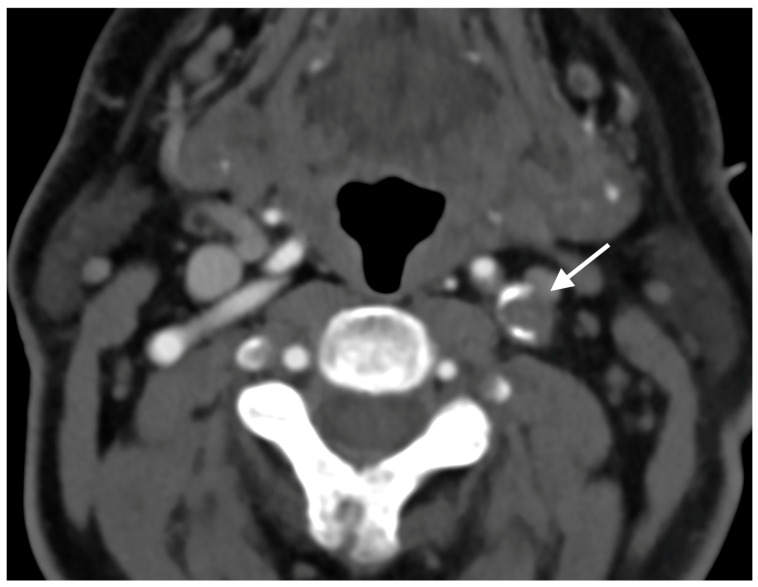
Initial CTA demonstrating complete nonattenuation of proximal cervical segment of left ICA.
